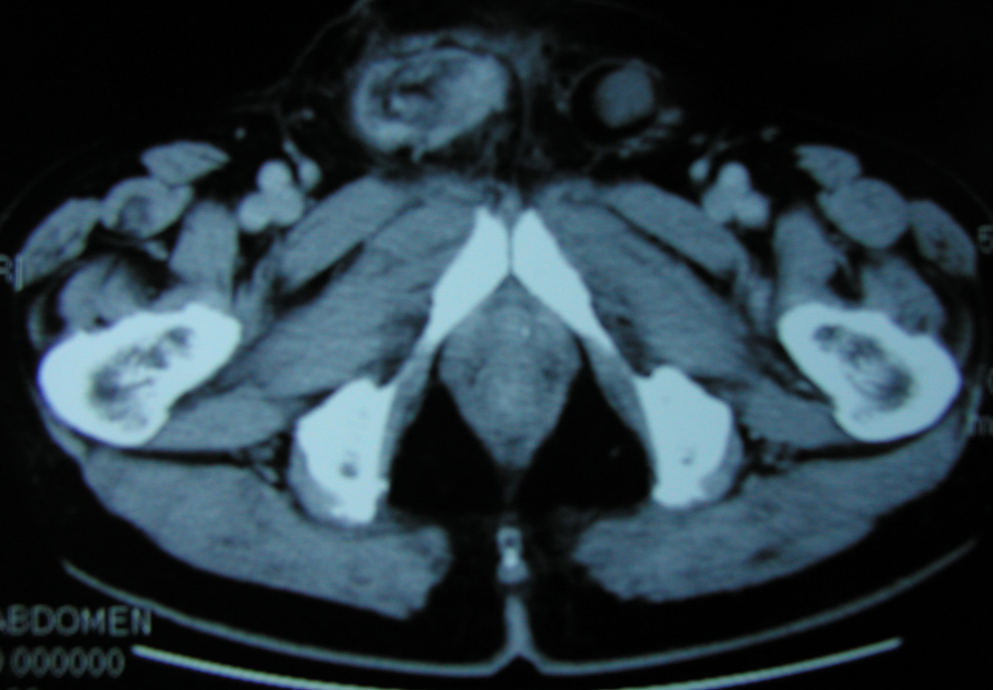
A heterogenous hypodense lesion with adjacent fat stranding in the right inguinal canal was noted on the CT scan.
